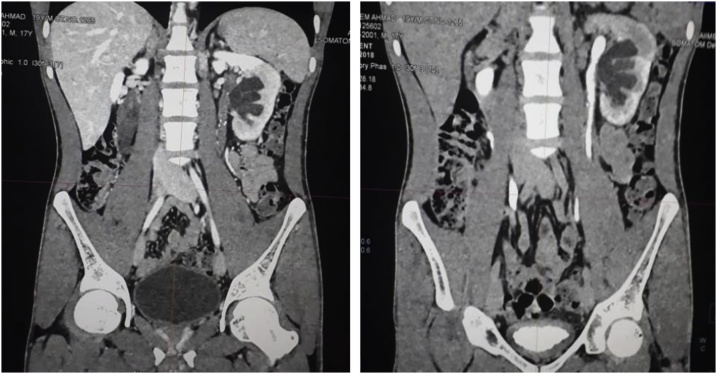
Left, contrast enhanced CT showing hypodense middle and lower pole renal parenchyma. Right, pelvicalyceal system corresponding to middle and lower pole kidney not communicating with ureter.
